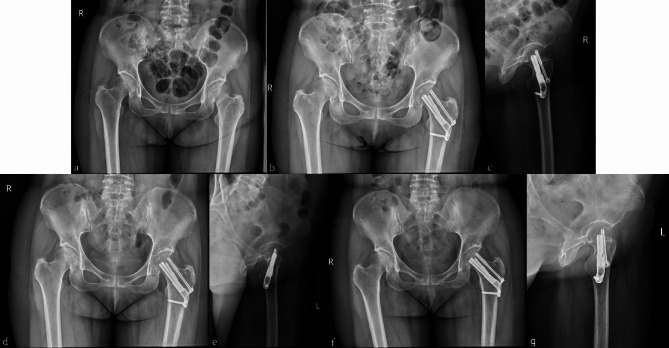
A 53-year-old female patient with left femoral neck fracture was treated with femoral neck systems combined with a cannulated screw (FNS + CS). (a) Preoperative anteroposterior X-ray image. (b,c) Postoperative anteroposterior and lateral radiographs revealing satisfactory reduction of the fracture and satisfactory location of the FNS + CS. (d,e) Anteroposterior and lateral radiographs taken 3 months after the surgery showed that the fracture had healed well. (f,g) Anteroposterior and lateral radiographs at 5 months follow-up showed no femoral neck shortening and screw withdrawal, and the neck-shaft angle was maintained well
